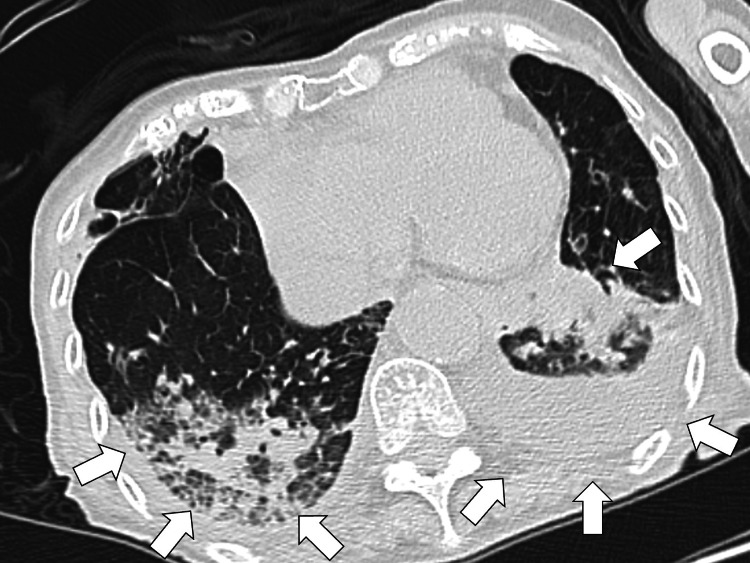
The chest CT revealing multiple infiltrates and ground-glass opacities in both lungs CT: computed tomography
